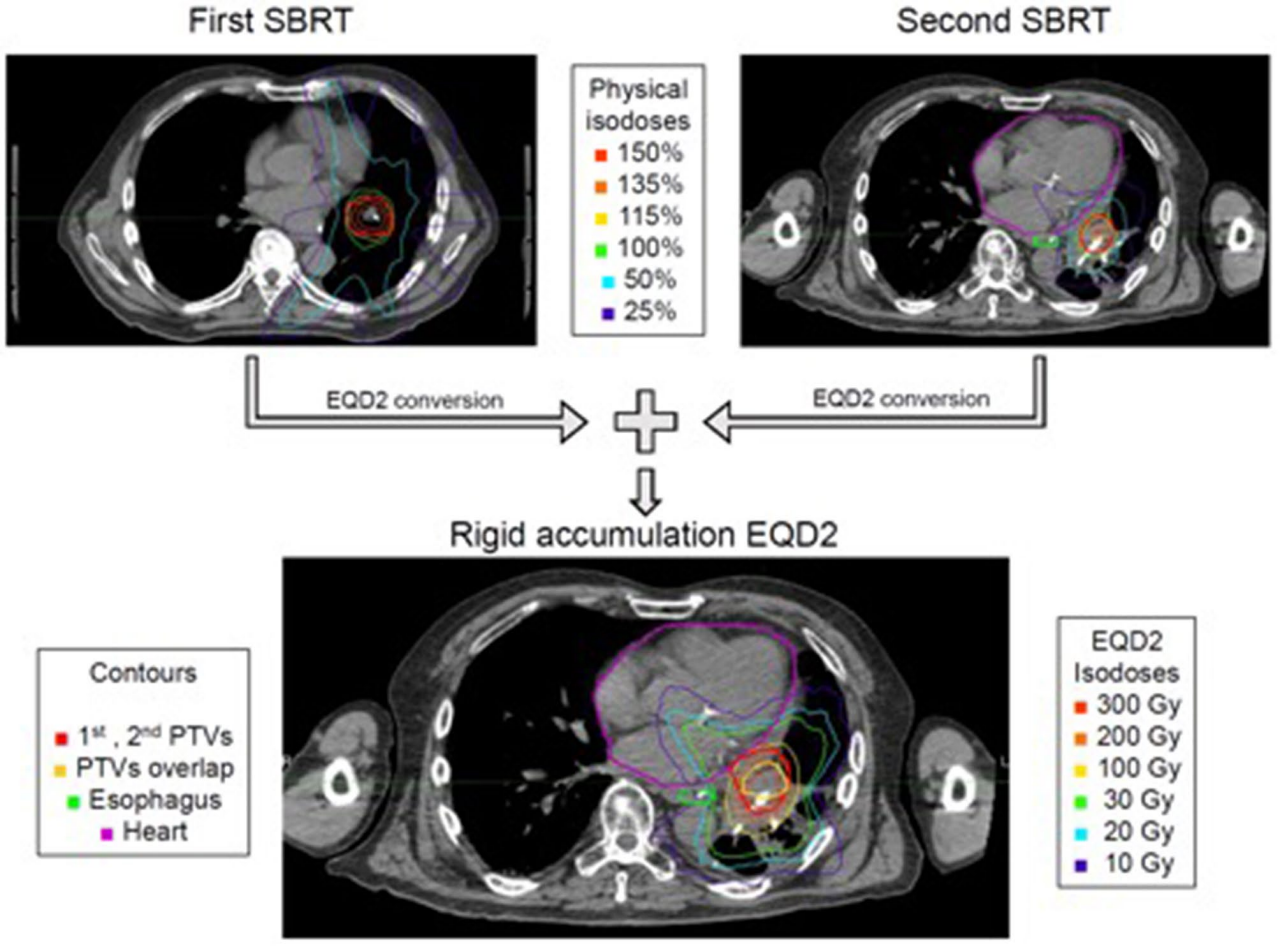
Exemplary case of the plan summation in MIM (version 6.9.2, MIM Software Inc., Cleveland, USA). The top row shows the first and second SBRT plans. The physical doses are normalized to 100% for the prescription to the PTV. Such doses are converted into EQD2 and rigidly accumulated onto the most recent CT. The result is shown at the bottom.
